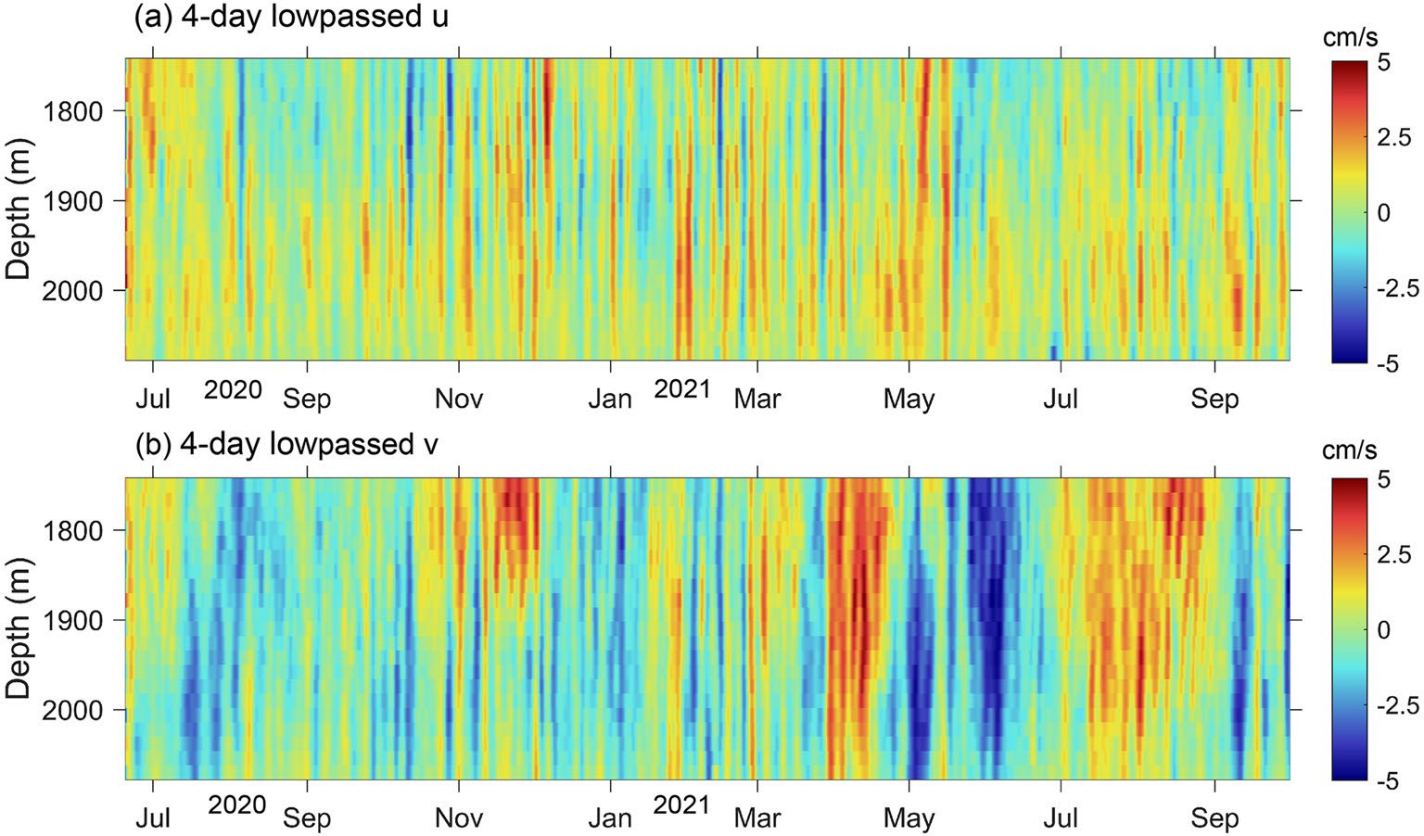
Observed horizontal velocity at the mooring TJ-T in the southern SCS. Time series of 4 days lowpassed zonal velocity u (a) and meridional velocity v (b) profiles from Acoustic Doppler Current Profiler (ADCP) observations.
